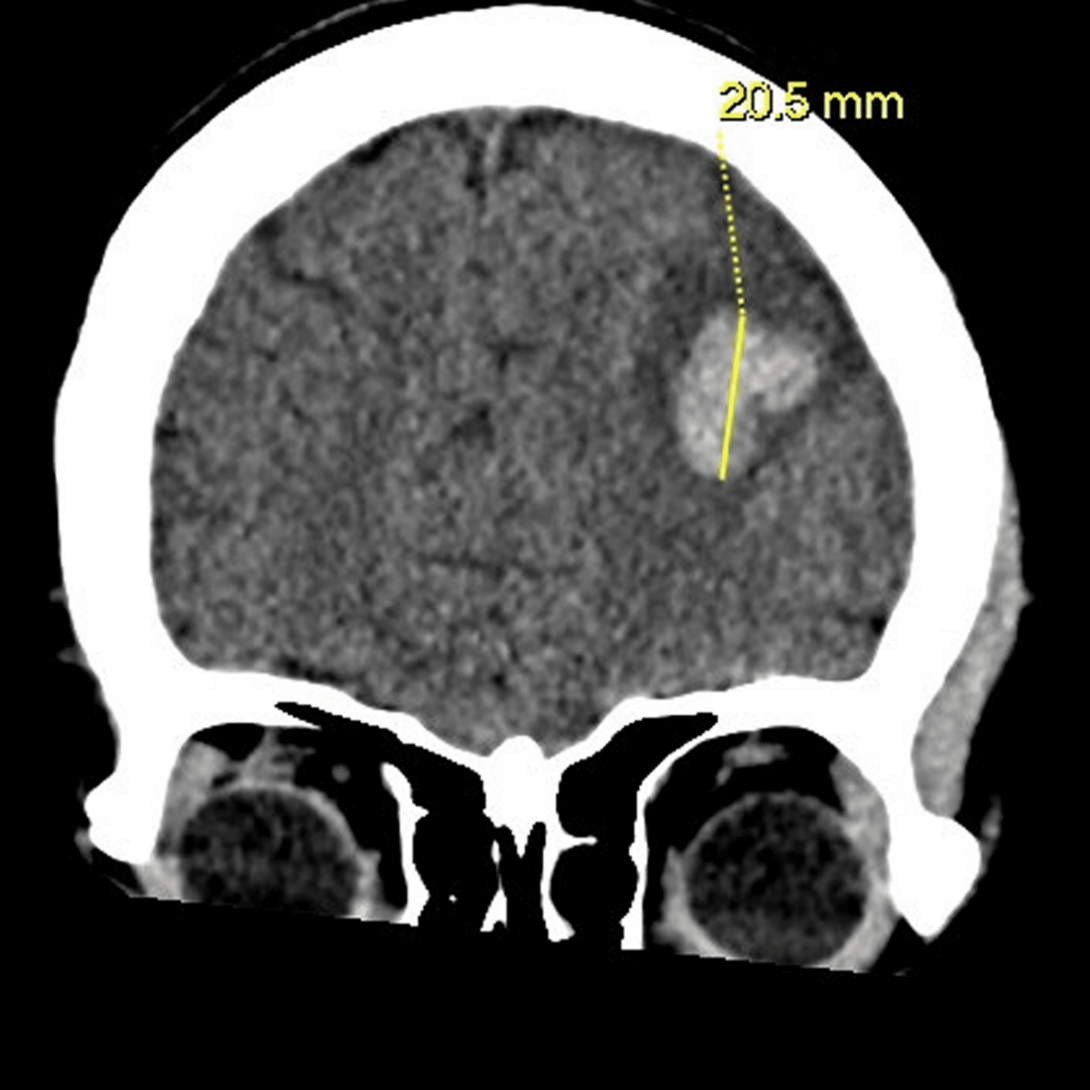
Coronal CT head imaging showing the area of brain haemorrhage
